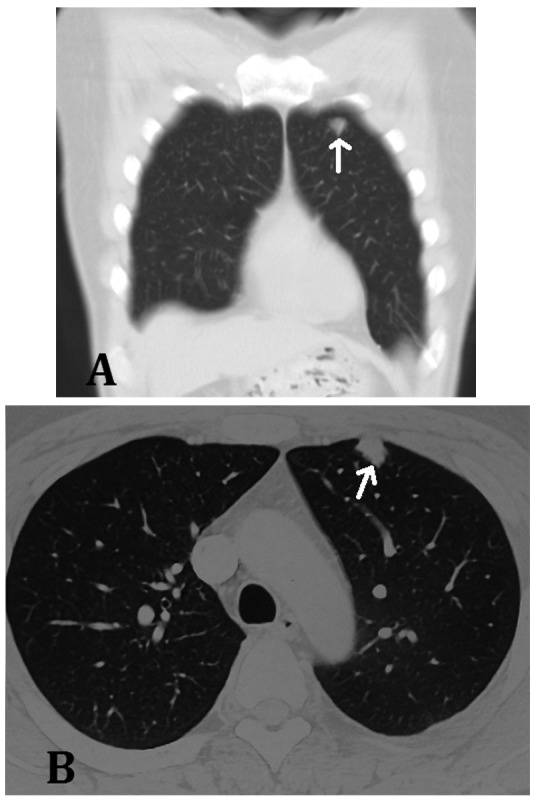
Case one. (A) Coronal and (B) transverse computed tomography scans of the chest, performed four months after the first surgery at the follow-up visit, showing a nodule with clear borders in the anterior upper left lobe of the lung.
